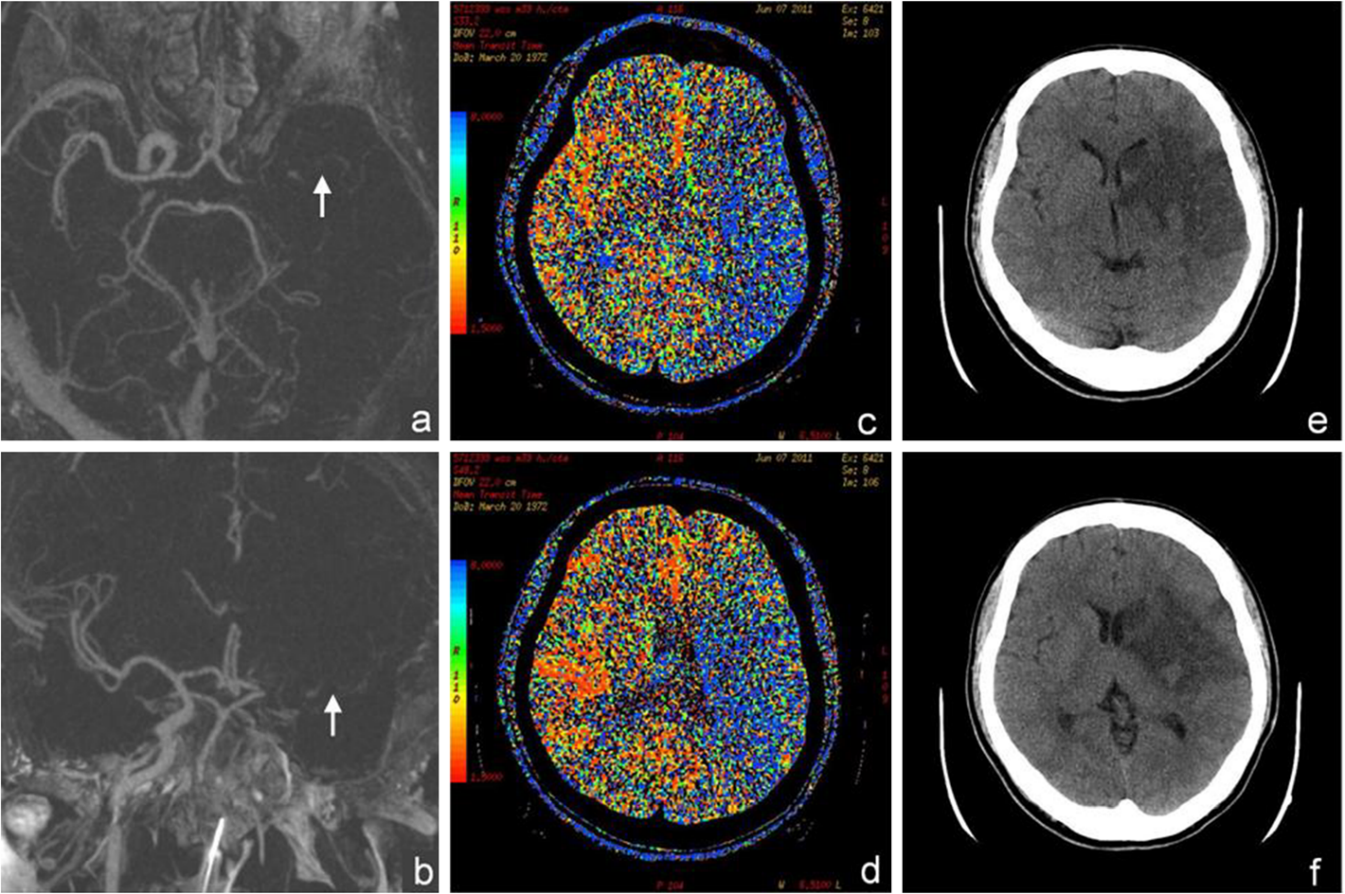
CT imaging of the patient. (a, b) Reconstructed CT angiography revealed nearly total occlusion of left proximal middle cerebral artery (marked by the arrows). (c, d) CT perfusion study showed prolonged mean transit time (the blue area indicates the area of prolonged transit time) in most of the left cerebral hemisphere, which indicated reduced perfusion. (e, f) Follow-up non-contrast CT one day after the stroke showed a hypodensity in the left basal ganglia and peri-sylvian area, suggestive of a recent infarct. The size was much smaller than the previous perfusion defect.
